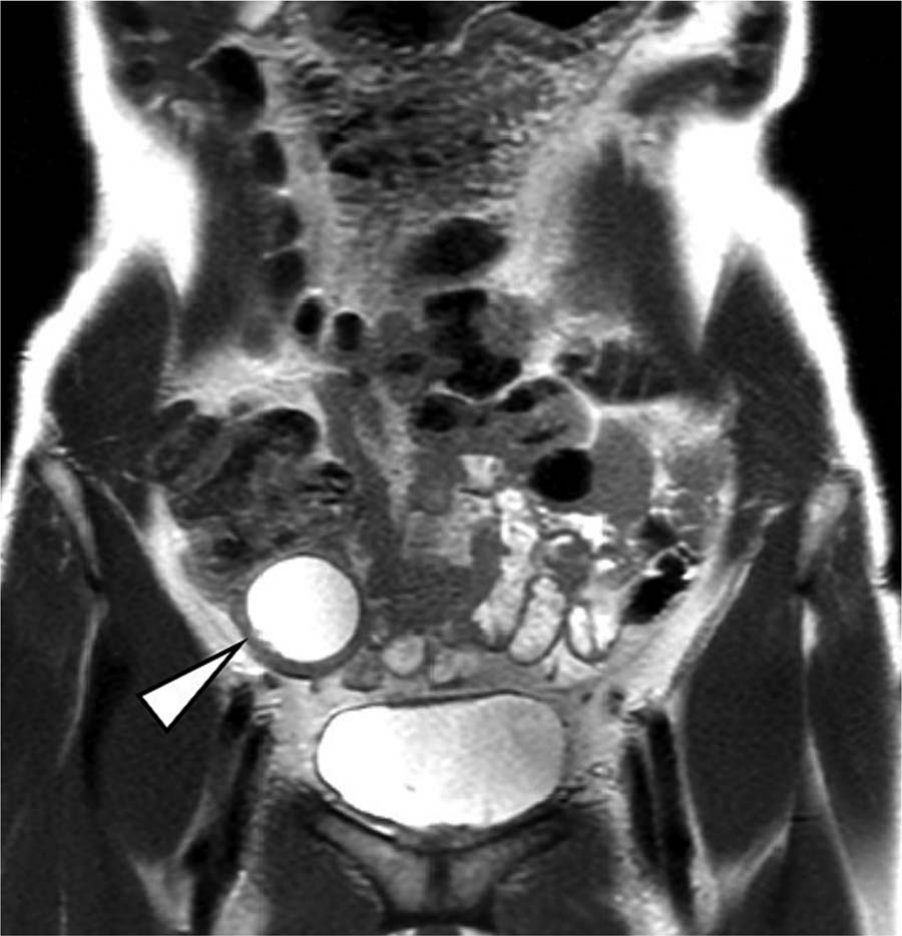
MRI image. The arrow indicates a well-circumscribed cystic lesion in the ileocecal region without mural nodules or solid components.
